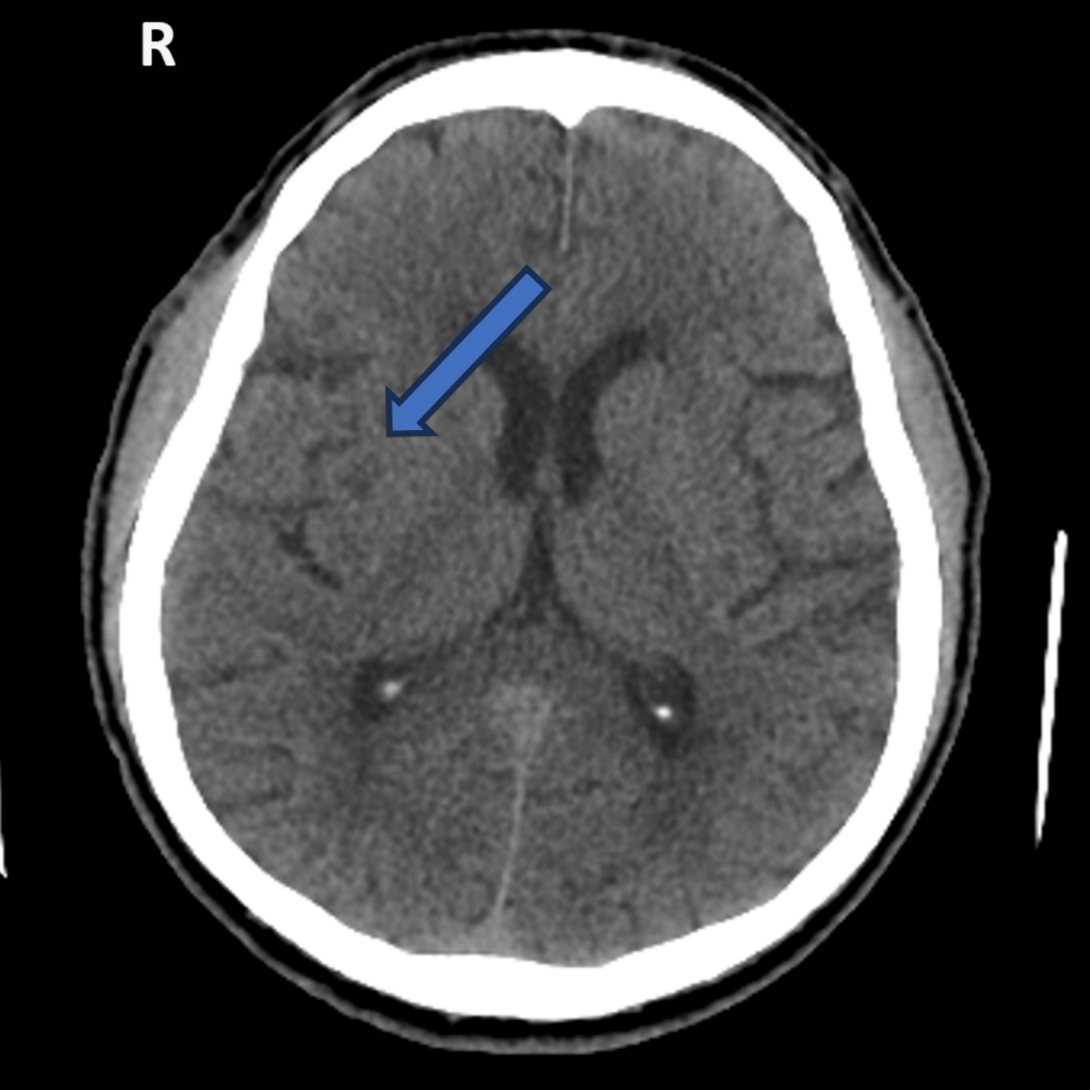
Twenty-four-hour repeat head CT scan. Axial view showing the right lenticuloradial hypodensity, consistent with recent ischemic stroke.
